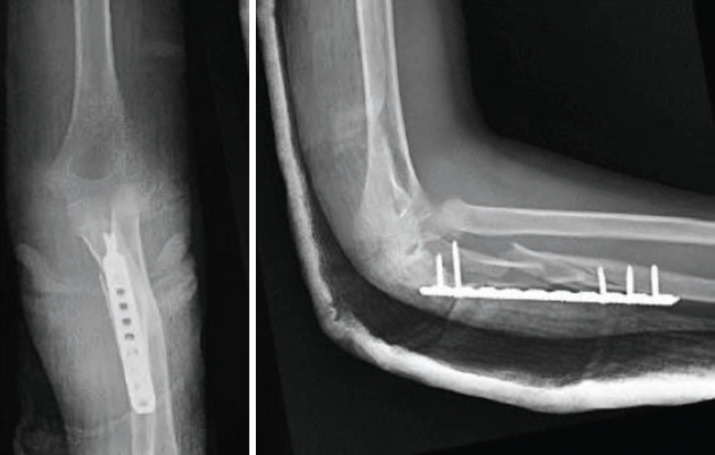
Post-operative radiographs at two weeks demonstrating satisfactory alignment of the ulnar shaft with internal fixation using bridging plate and screws. The radial neck fracture was adequately reduced, and there was no displacement of the distal radial fracture.
